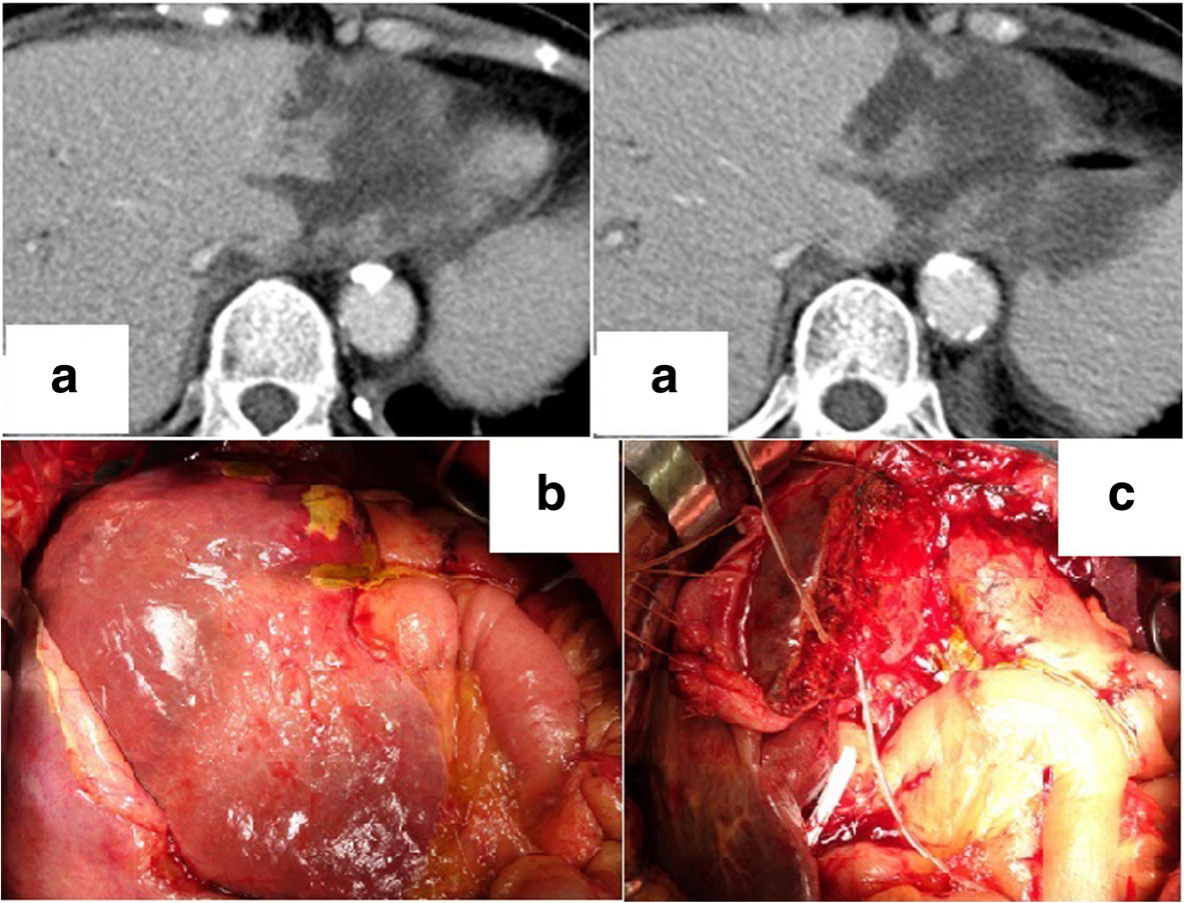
Case 2 CT findings 5 days after pancreaticoduodenectomy and relaparotomy findings. a Extensive necrosis is observed in the lateral segment of the liver. b The lateral segment becomes necrotic and is widely covered with yellow coat. c Lateral segmentectomy with external biliary drainage is performed
